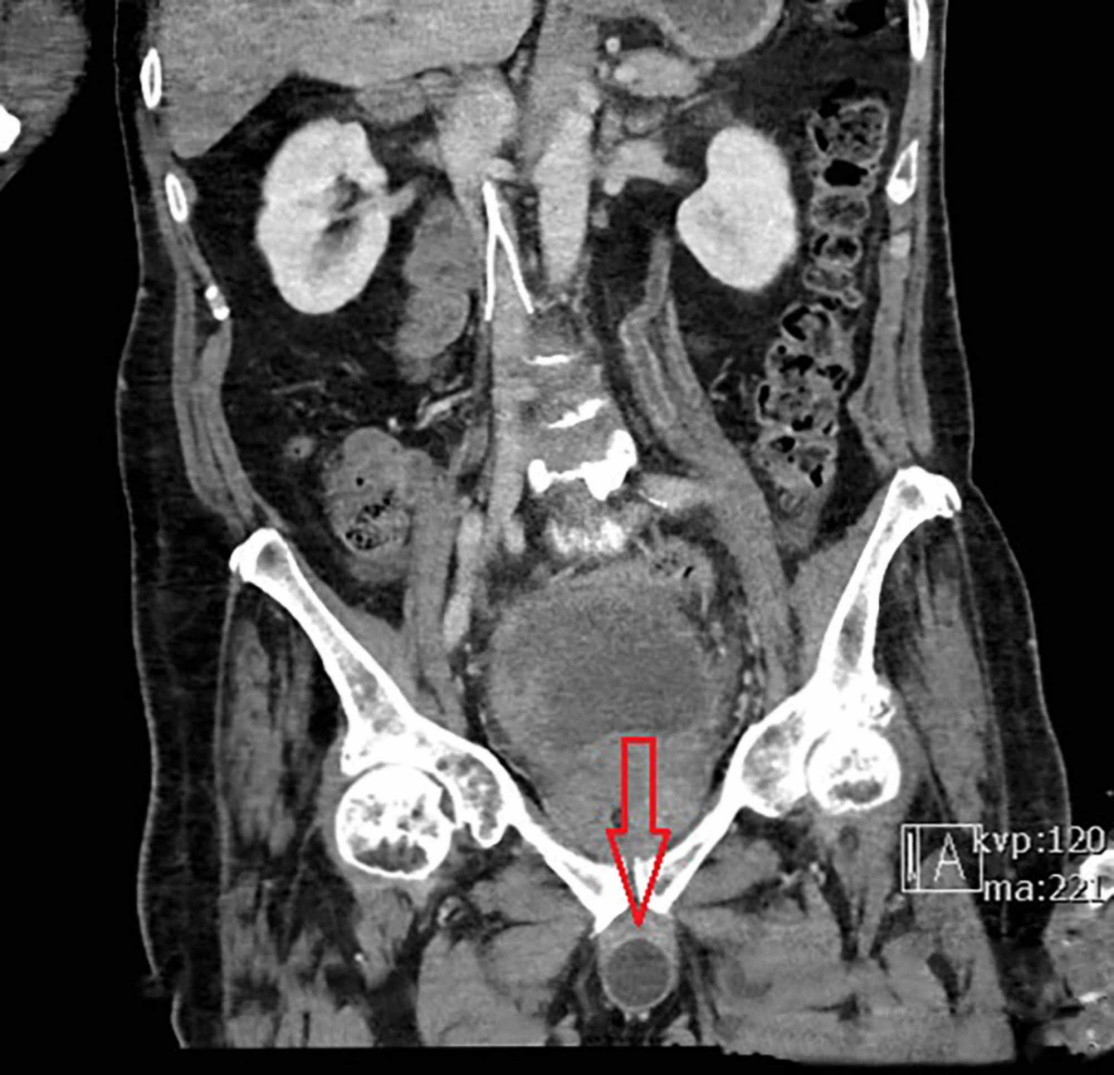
Coronal CT scan with the arrow indicating inflated catheter in penile urethra.
